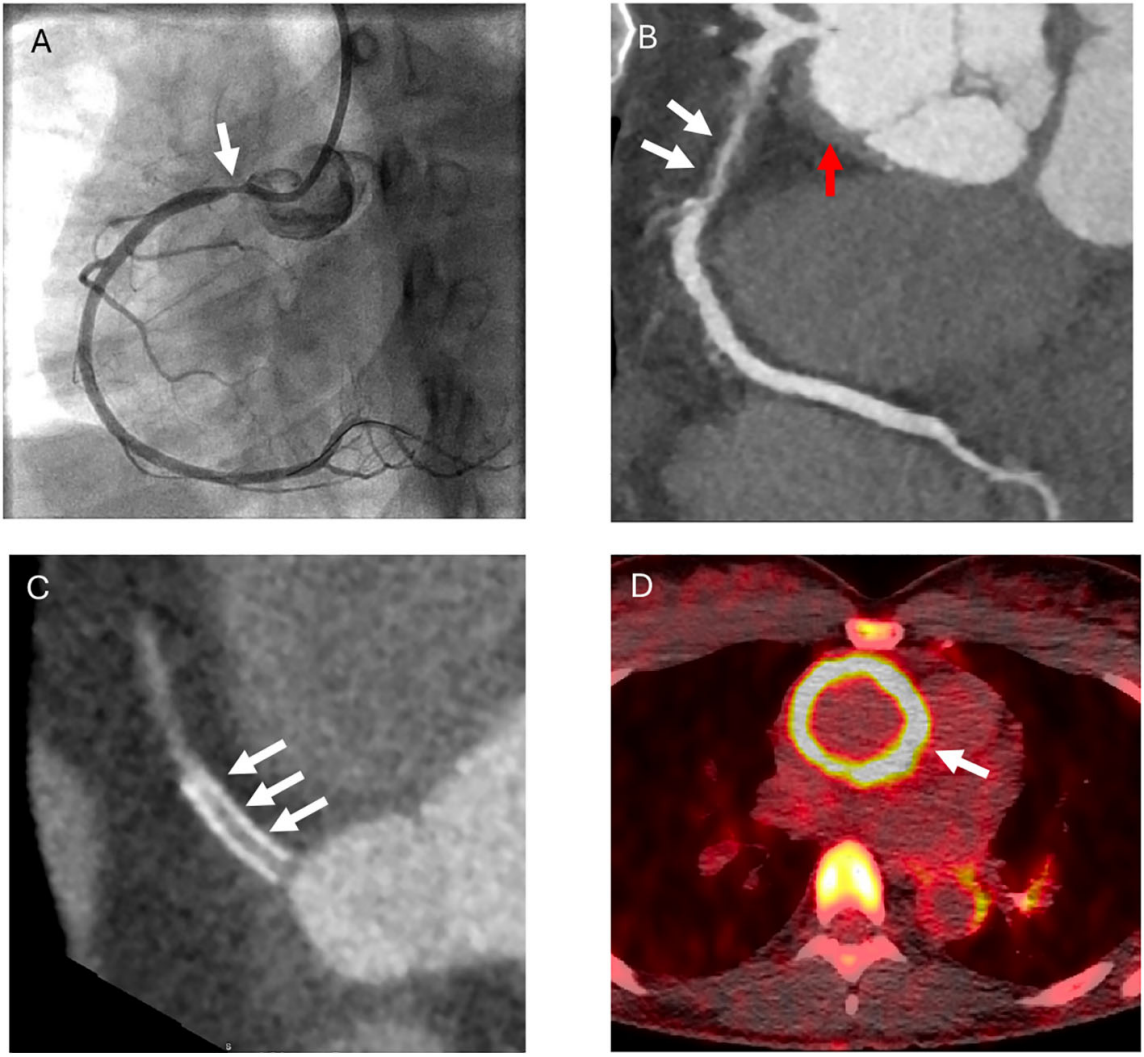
Representative images of coronary artery vasculitis in Takayasu’s arteritis. Catheter based angiography demonstrating ostial vasculitic lesion in the right coronary artery (A). Cardiac computed tomographic angiography showing stenotic proximal right coronary artery with associated wall thickness (white arrows) and wall thickness of the aorta (red arrow) (B). Cardiac computed tomographic angiography showing in-stent restenosis in the right coronary artery (C). Positron emission tomography/computed tomography showing increased FDG radiotracer in the ascending aorta in a patient with concomitant active coronary arteritis (D). .
